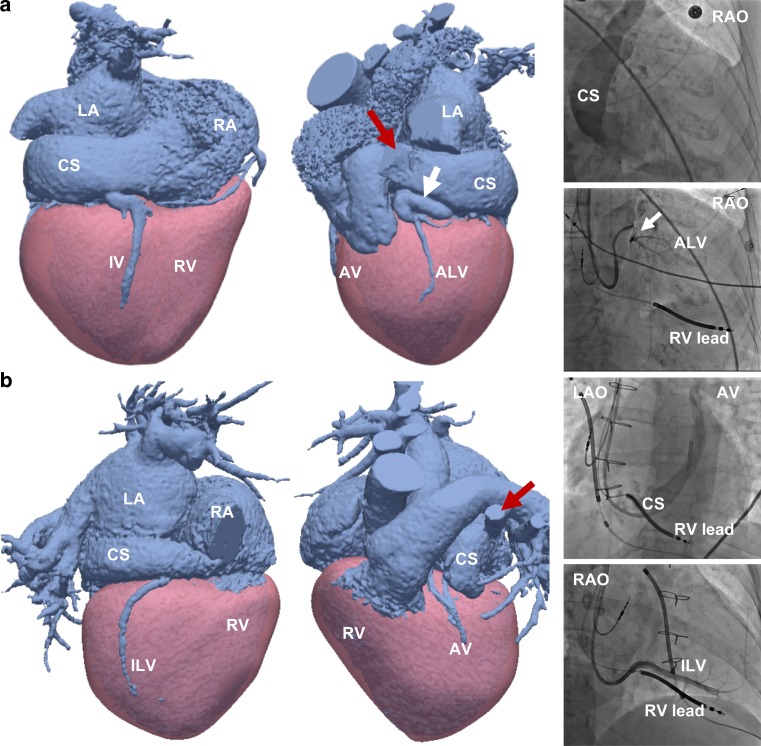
CTA and FA of a 77-year-old woman (patient 4, a) and a 49-year-old woman (patient 10, b) with a left-sided persistent superior vena cava (*SVC*, *red arrow*) and CS dilatation. Note the sharply angulated vein (*white arrow*) in patient 4 (a) and the limited coronary venous anatomy in patient 10 (b*)*. *ALV* anterolateral vein, *AV* anterior vein, *CS* coronary sinus, *ILV* inferolateral vein, *IV* inferior vein, *LA* left atrium, *LAO* left anterior oblique, *RA* right atrium, *RAO* right anterior oblique, *RV* right ventricle
